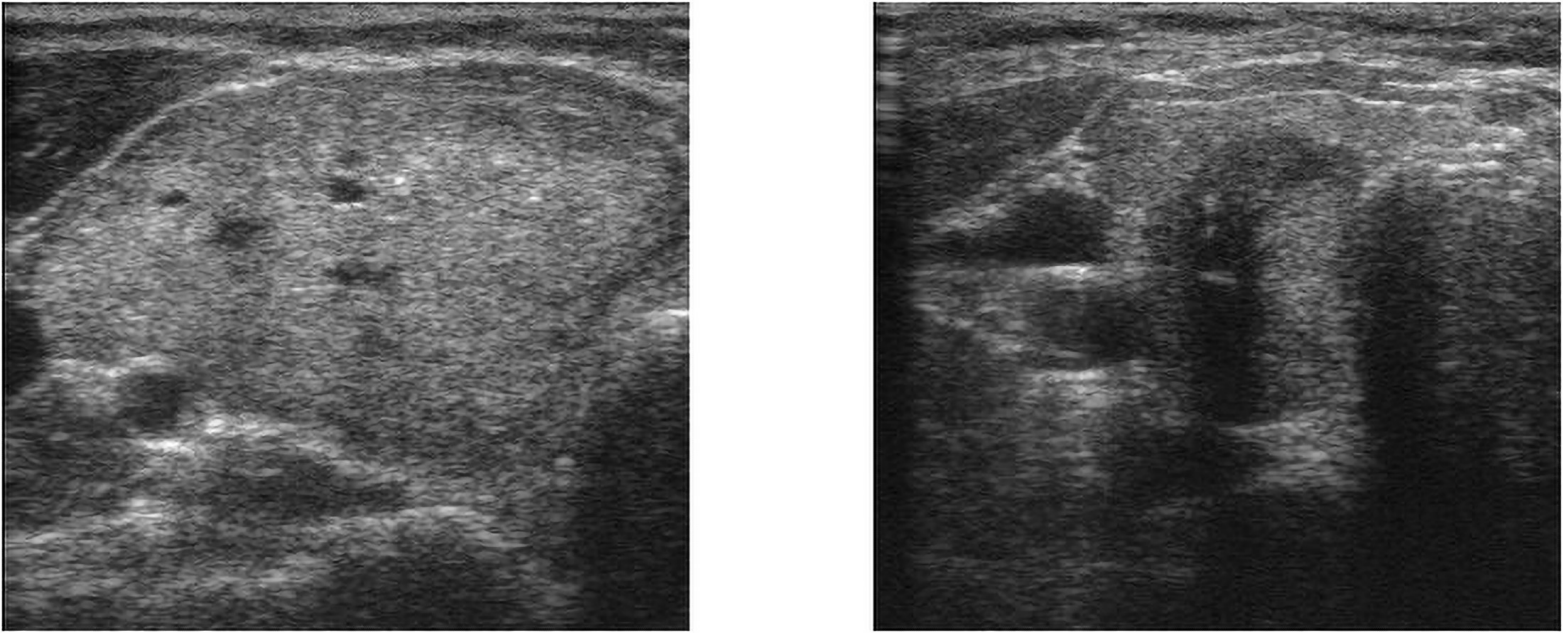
Ultrasound images from DDTI: TIRADS 2 on the left-side with well defined margins and no calcification, labeled as “benign”; TIRADS 5 on the right-side with micro-calcification, labeled as “malignant”.
